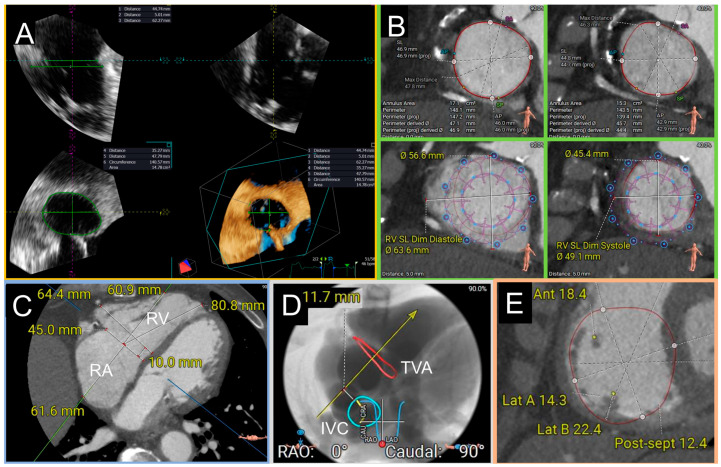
Screening for TTVR, key imaging features. Panel (A): TEE 3D volume of TV to assess TV annulus (diameters and perimeter) and basal RV diameter. Panel (B): CT evaluation of TV annulus (upper panels) in diastole (panel (B) top left) and systole (panel (B) top right), and RV at basal level in diastole (bottom left) and systole (bottom right); Evoque anchors are represented in light blue. Panel (C): working room assessment; RA and RV length are measured from a reformatted 4 chamber view. Panel (D): fluoroscopic reconstruction of implant projection derived from CT images; the distance between inferior vena cava and TVA centerline is measured; in this case it was 11.7 mm (>25 mm is considered a cut-off for non-feasibility). Panel (E): contrast CT, evaluation of position and distance of papillary muscles. CT computed tomography; IVC: inferior vena cava; RA: right atrium; RV: right ventricle; TEE: transesophageal echocardiography; TTVR: transcatheter tricuspid valve replacement; TV: tricuspid valve; TVA: tricuspid valve annulus.
